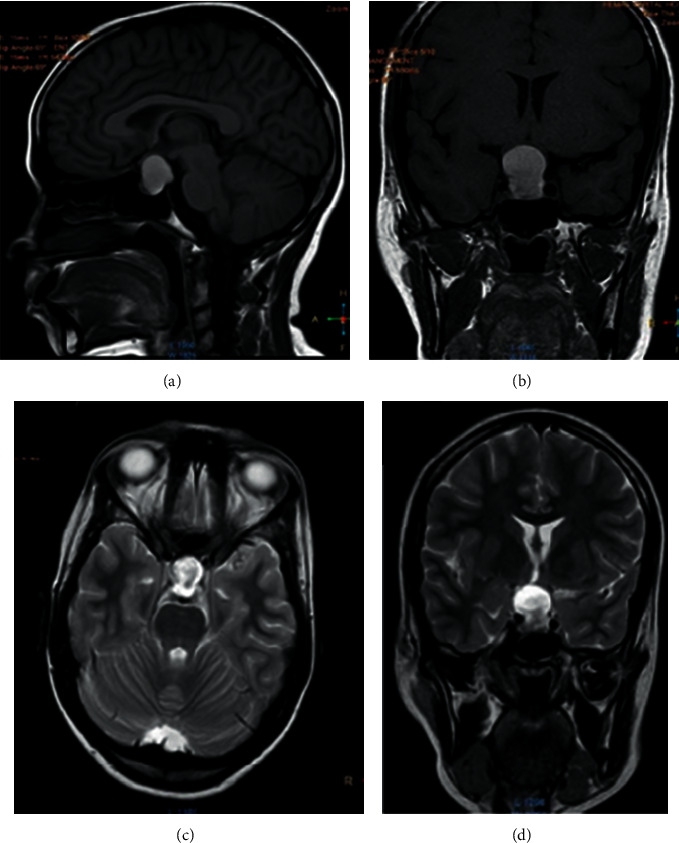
MRI of this patient showing lobular tumor occupying sellar and suprasellar region measuring 2.4  cm × 2.0 cm × 2.0 cm. Caudal part of the lesion showed intermediate signal intensity in T2-weighted images. Superior mass effect is seen on the optic chiasma, optic tract, and AI segments of bilateral anterior cerebral arteries. Mass is bulged into the sphenoid sinuses. ((a, b)) T1-weighted, gadolinium-enhanced sagittal and coronal views. ((c, d)) T2-weighted axial and coronal views.
